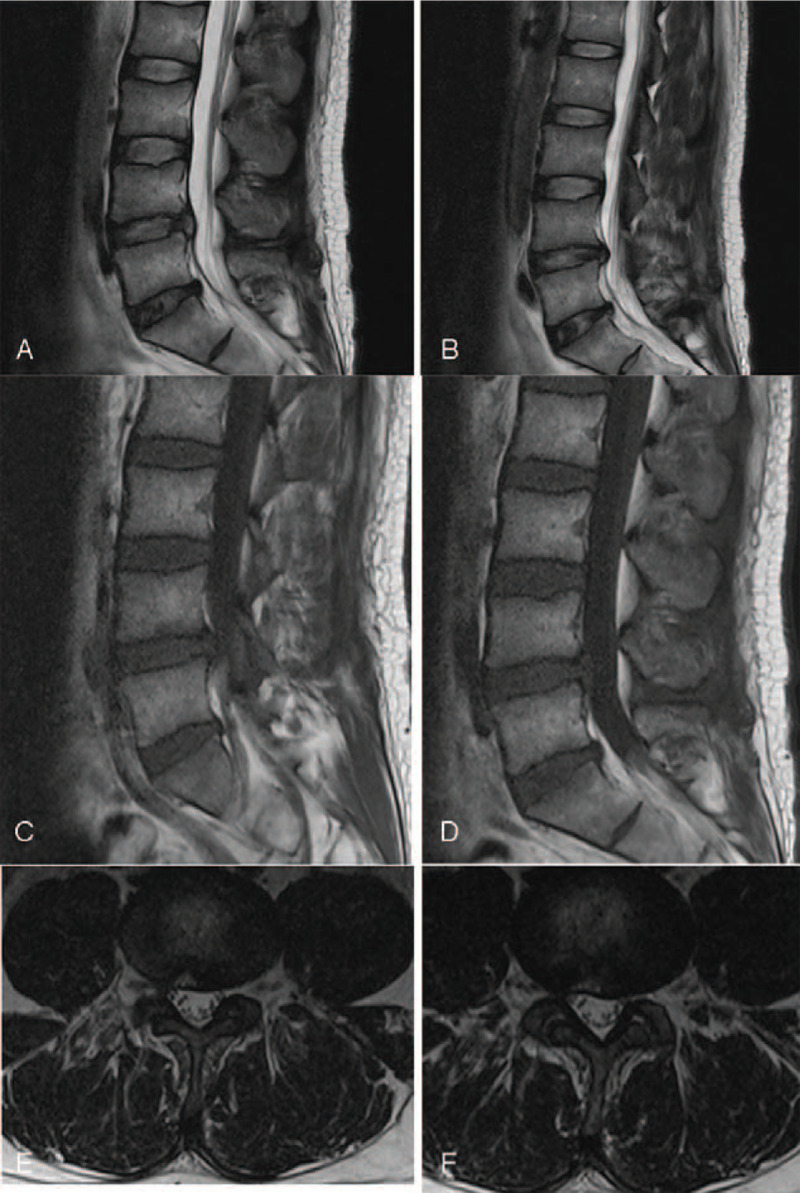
MR imaging at 3 mo after surgery revealed the disappearance of cystic mass that was found originally at L4/5 level on the right side, the cystic long T2 signal at the posterior part of the L4–5 disc, and also the herniated disk at the level of L4/5. (A and B) Sagittal T2WI; (C and D) sagittal T1WI; (E and F) cross-sectional scanning.
